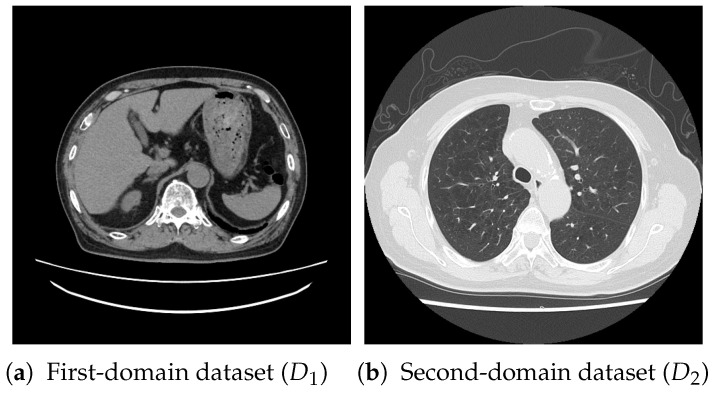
Examples of chest CT images on the subsets from the J-MID database: (a) first-domain dataset ($D_{1}$) and (b) second-domain dataset ($D_{2}$).
